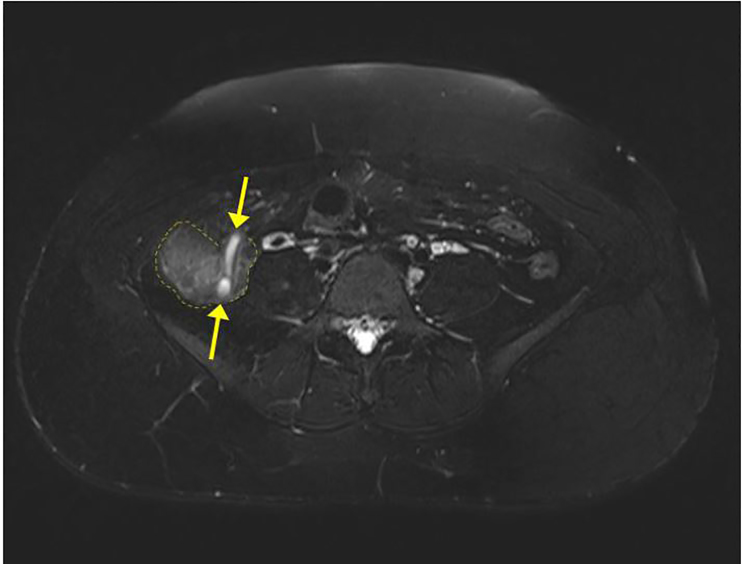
Axial T2-weighted magnetic resonance image demonstrates a dilated appendix (demarcated by yellow arrows) with increased signal of surrounding fat indicating inflammation (outlined by dashed yellow lines).
